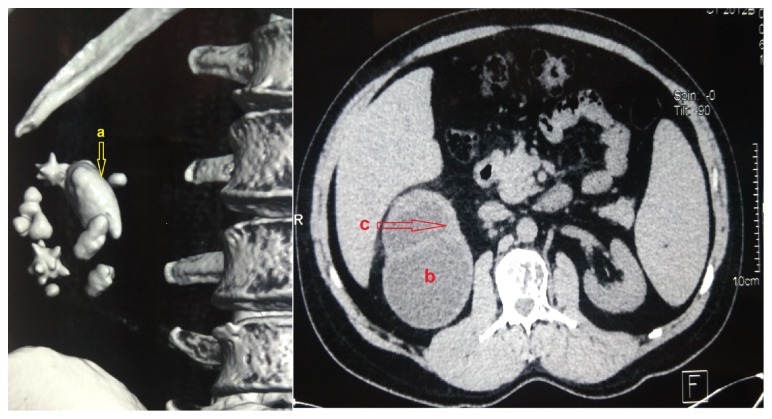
CT scan demonstrating large renal calculi and upper pole hydronephrosis: (a) large right renal pelvic calculi; (b) upper calix hydronephrosis; (c) thinned surrounding renal cortex.
